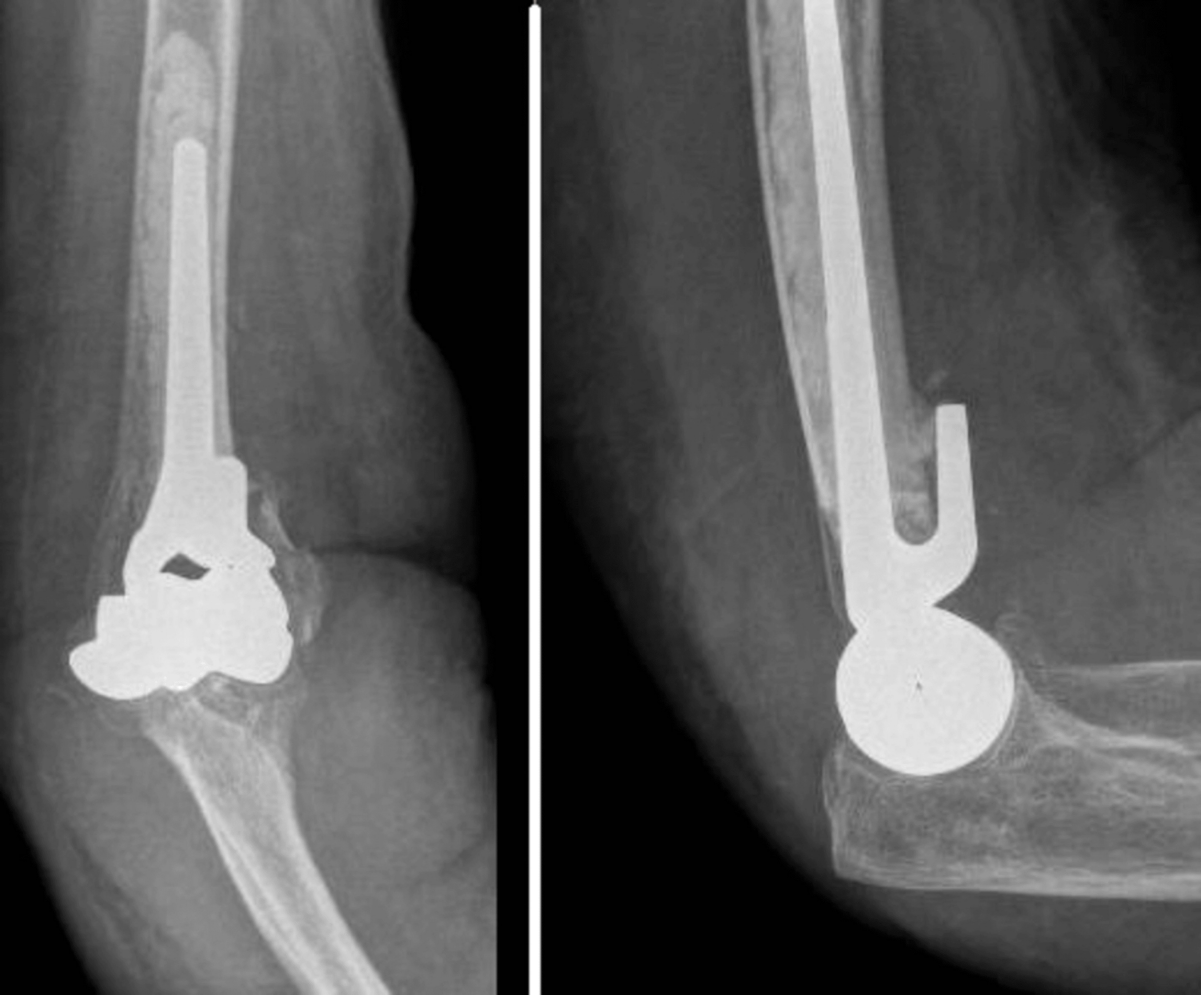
Postoperative radiograph of a patient's elbow after DHH. DHH: distal humerus hemiarthroplasty
